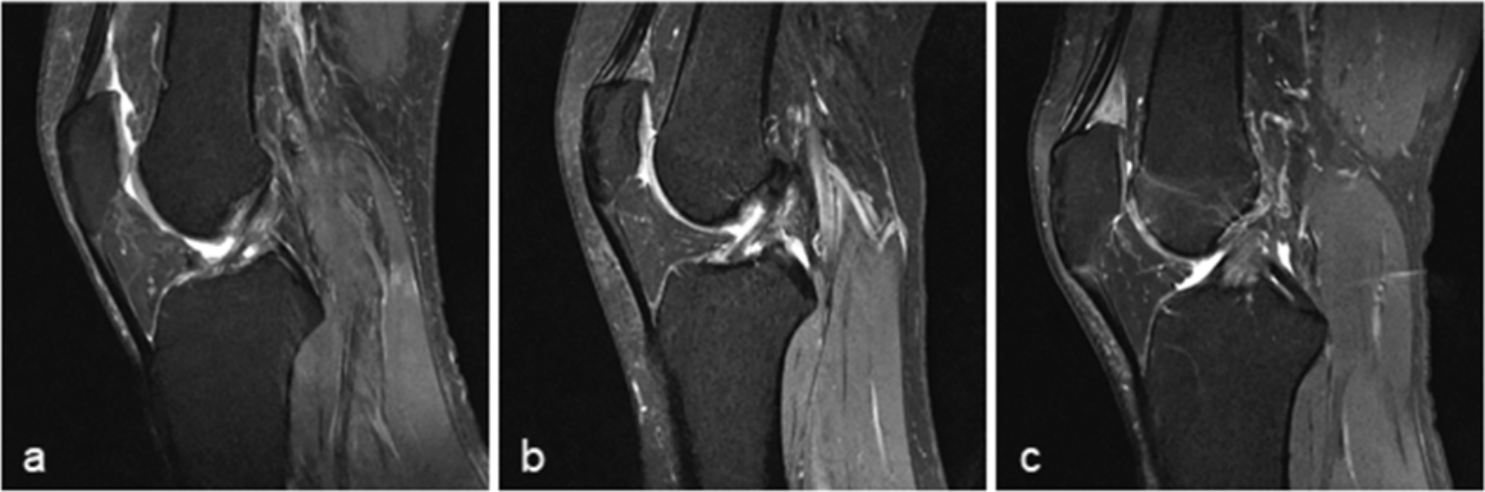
Suprapatellar quadriceps fat pad edema (QFPE). Sagittal magnetic resonance imaging of the knee shows the QFPE scoring system for a normal; b mild or intermediate, grade 1; and c severe or grade 2 QFPE.
